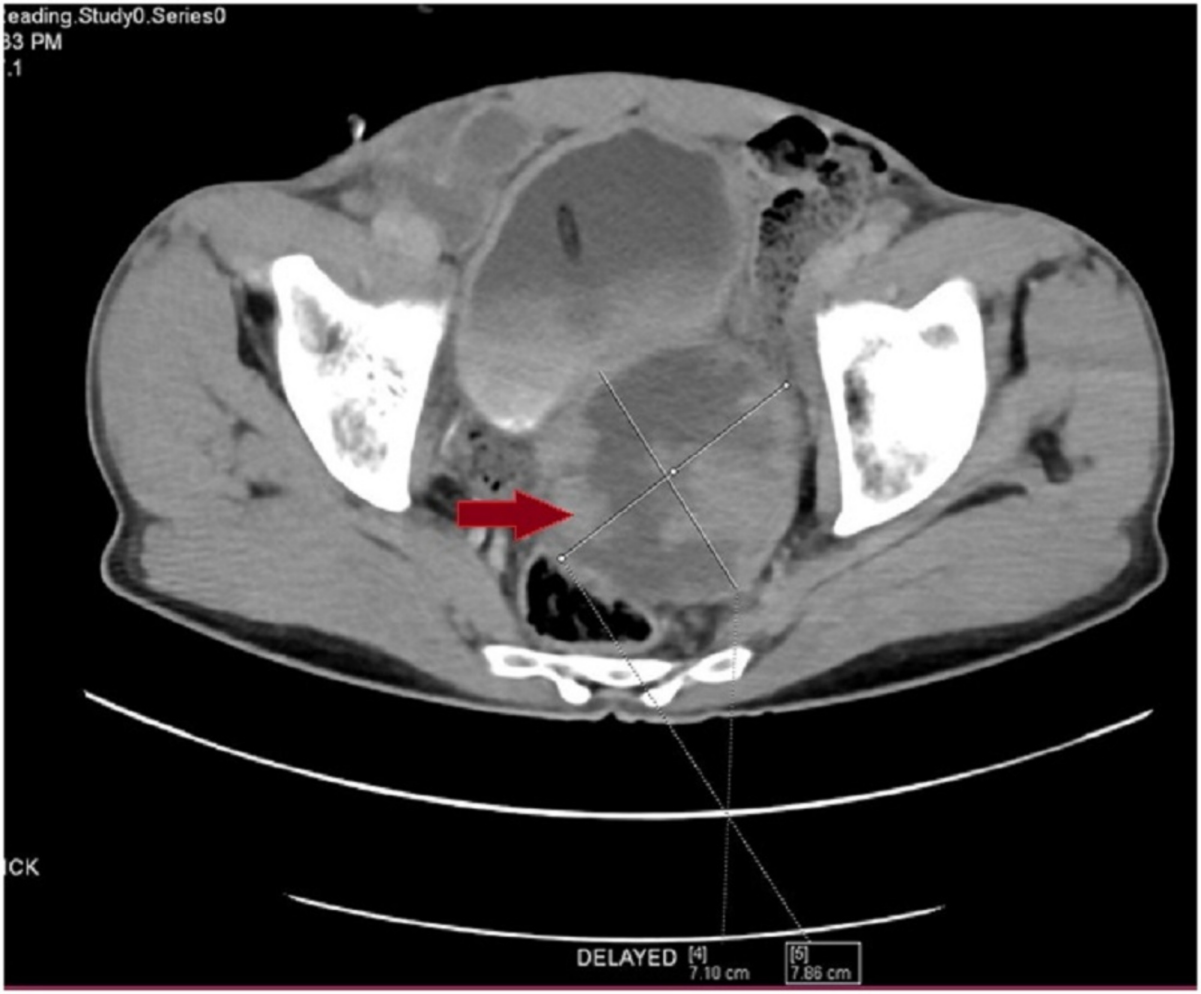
Contrast-enhanced computed tomography with axial image of delayed phase showing large intra-prostatic cyst (red arrow)
